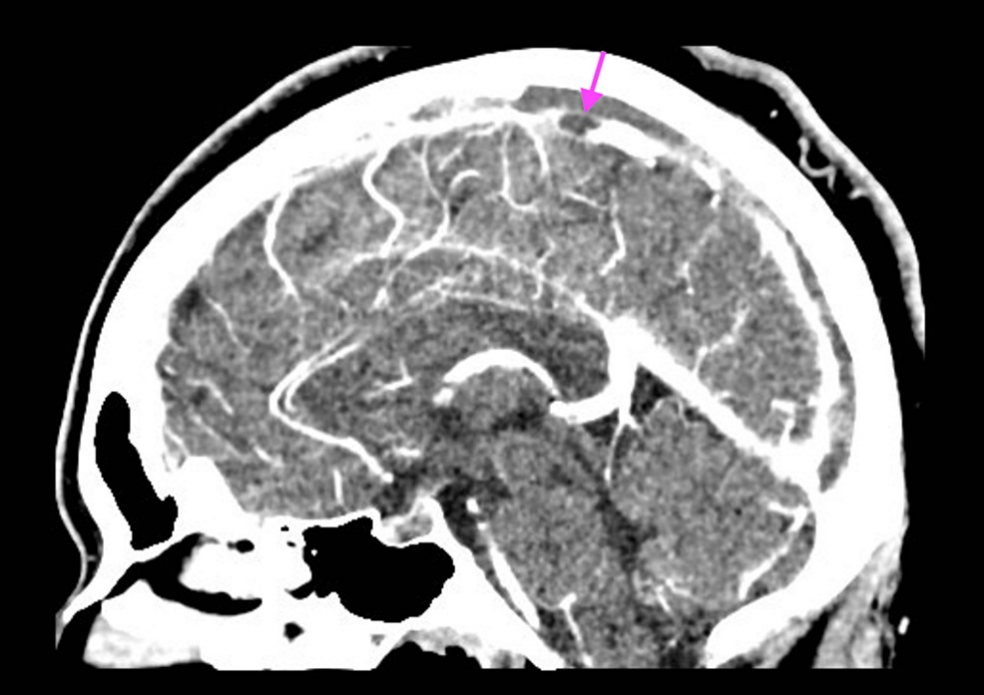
Acute venous dural sinus thrombosis present in the superior sagittal sinus seen on the CT venogram of Case 1
